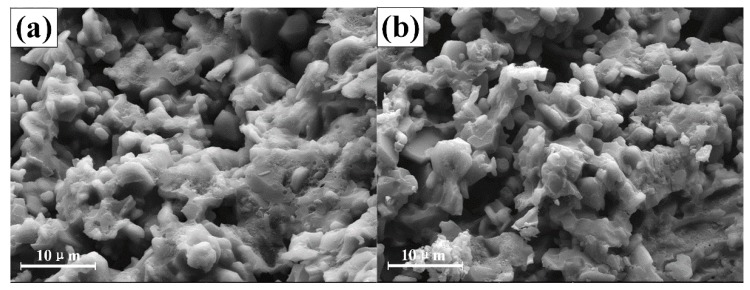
The microstructure of adhesive curing with the CT-1 (a) and CT-6 (b) temperature curve.
